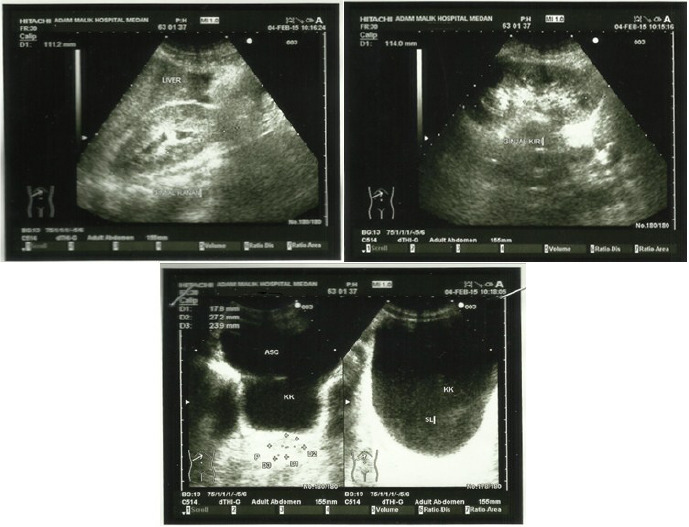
Renal and bladder ultrasonography
